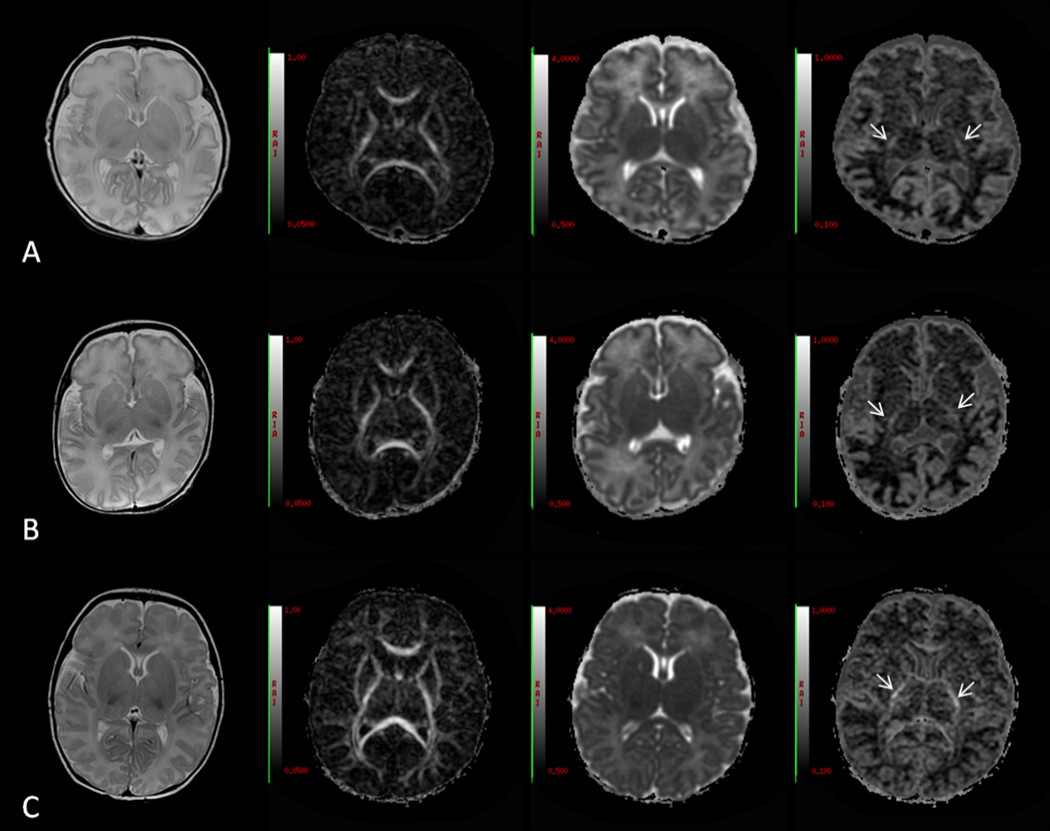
Conventional MRI and DKI of the preterm infants at different PMAs. Rows A to C represent PMAs of 34 weeks, 37 weeks and 40 weeks, respectively. The columns (left to right) represent the T2WI, FA, MD and MK maps. The RAI value range in the MD column was 0.5×10^-3^mm/s^2^~4×10^-3^mm/s^2^.The T2 signal intensity (SI) of the PLIC gradually decreased as PMA increased from 34 weeks to 40 weeks; meanwhile, the signal intensity on the FA and MK maps increased, as shown with a white arrow on the MK map. The image contrast between the GM and WM decreased on the T2WI and MD maps due to the decreased water content.
